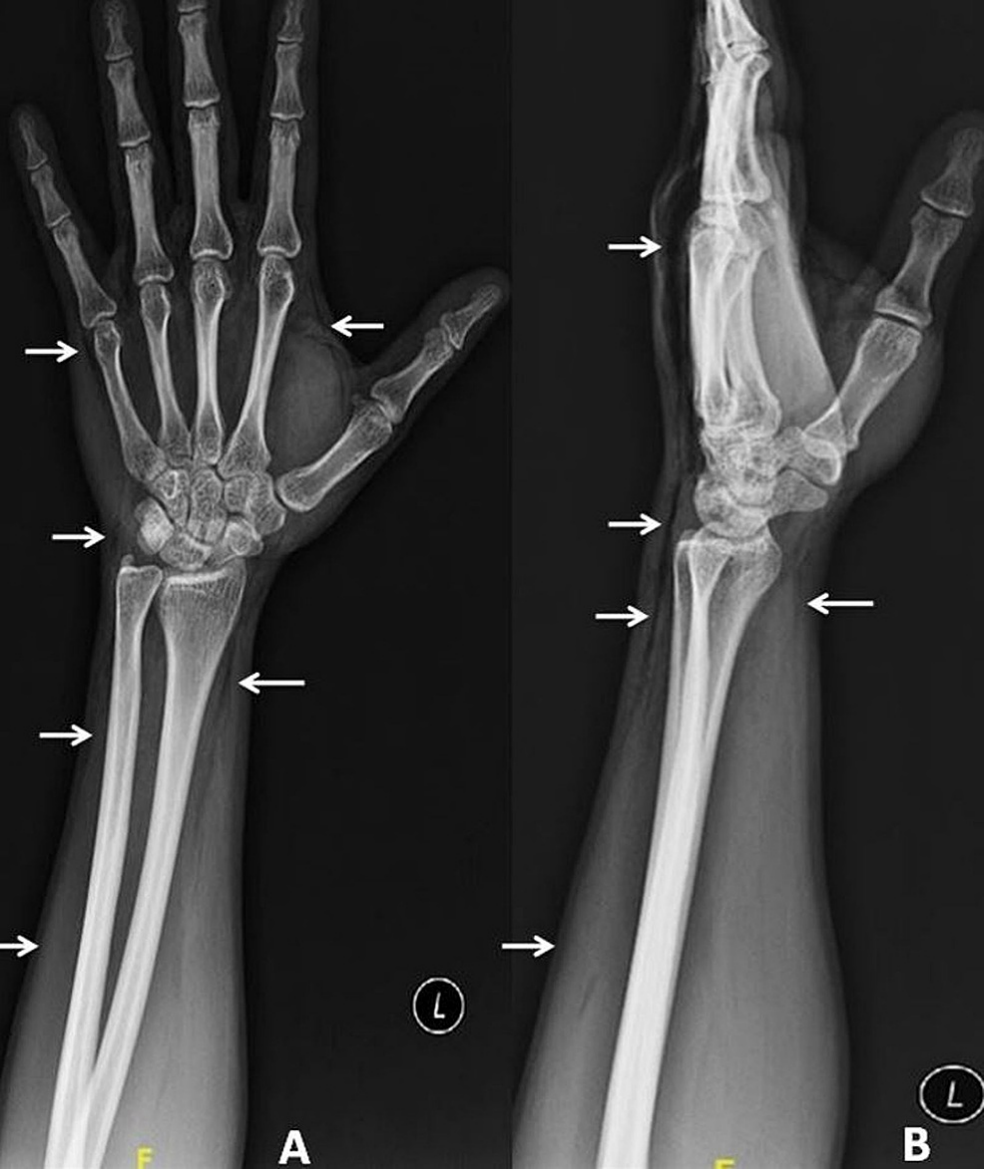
X-ray of the left upper limb showing subcutaneous emphysema A: anteroposterior view, B: lateral view
